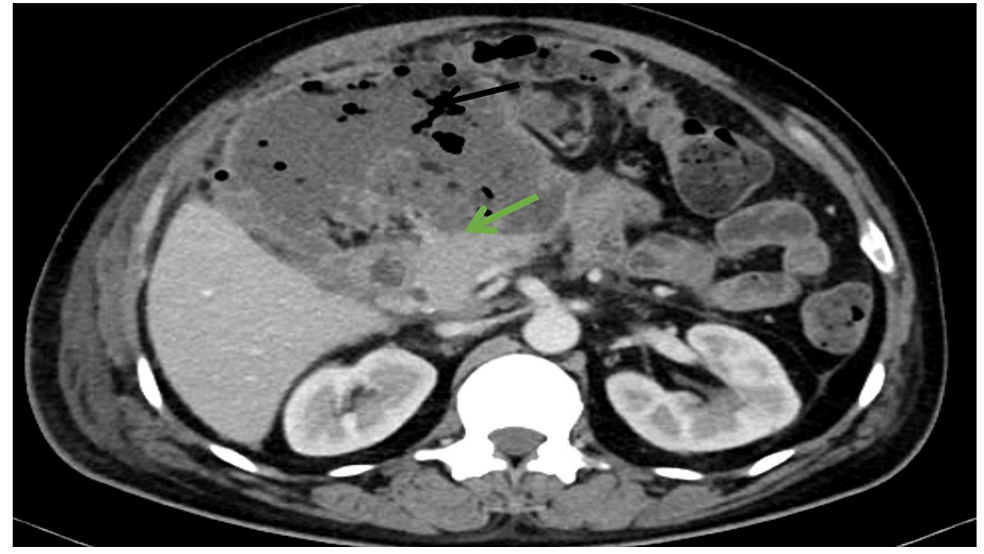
Contrast-enhanced computed tomography (CECT) image of patient in Case 1 demonstrating collection with air pockets (black arrow) adjacent to normally enhancing head of pancreas (green arrow).
